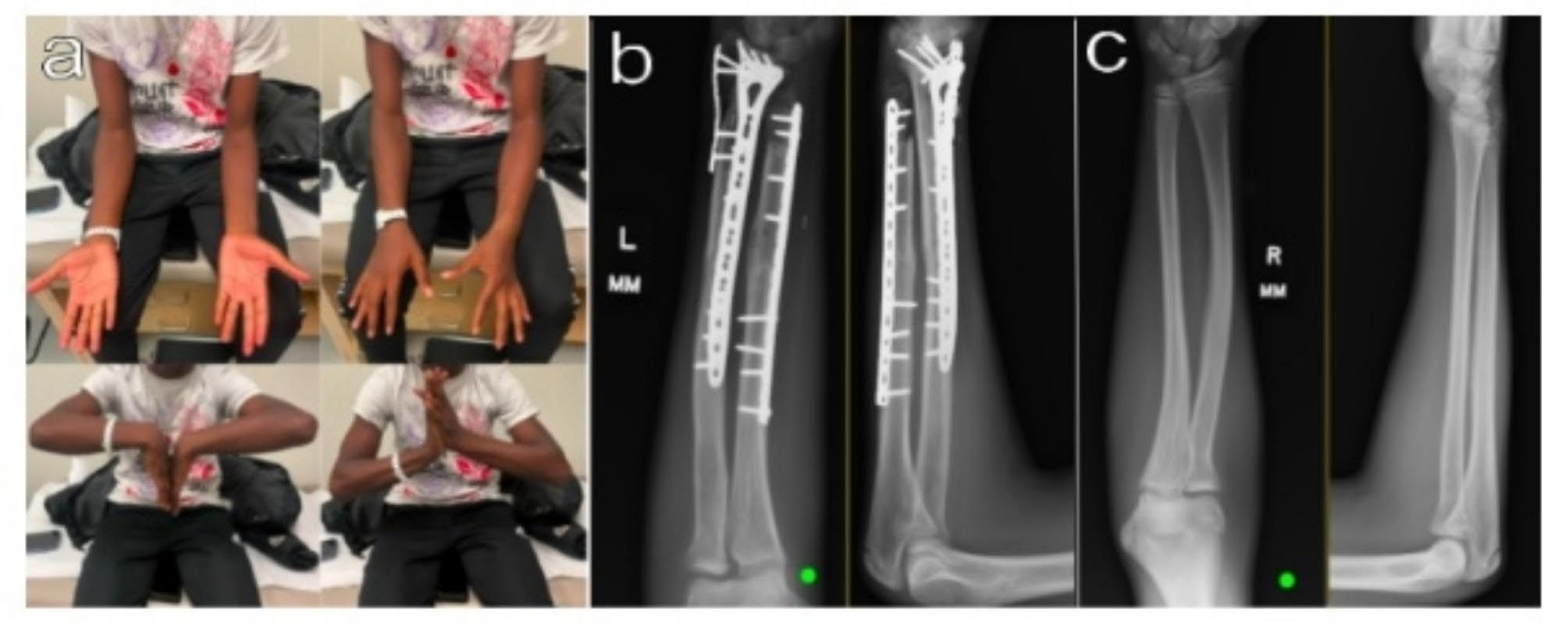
a-c: 6 months after surgical management. a Active range of motion with full supination of 90 degrees, 80 degrees of pronation, 83 degrees of flexion, and 75 degrees of extension. b Radiographs at six months show intact hardware and full incorporation of the radial allograft and continued incorporation of the ulnar allograft. c Radiographs of the contralateral forearm at the time of the patients 6 month postoperative appointment. These have been provided for reference to the amount of erosion the tumor caused to the left ulna
